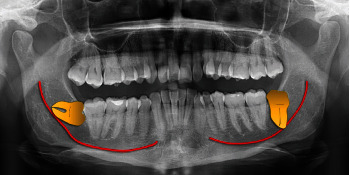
A radiographic example of the possible anatomical relationships between IAN and M3.
